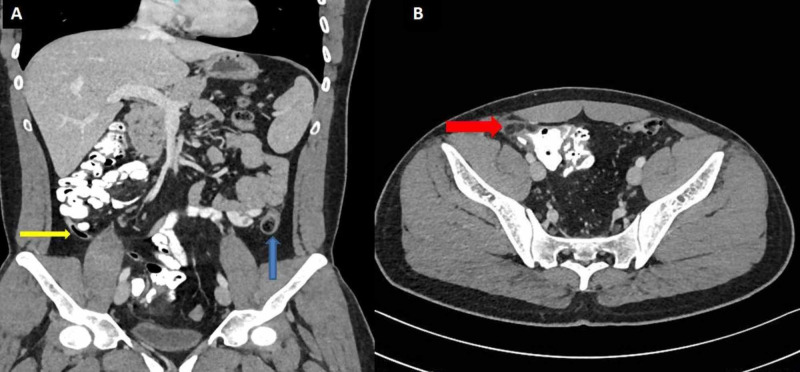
Contrast-enhanced CT abdomen and pelvis. A sagittal section of CT abdomen and pelvis shows normal appendix (yellow arrow) and a fat density oval-shaped lesion (blue arrow) anterior to the descending colon with perilesional fat stranding and a minimal amount of free fluid (A). An axial section of the CT abdomen and pelvis shows a second fat density structure (red arrow) anterior to the cecum with peripheral fat standing, free fluid, and adjacent cecal wall thickening (B).
